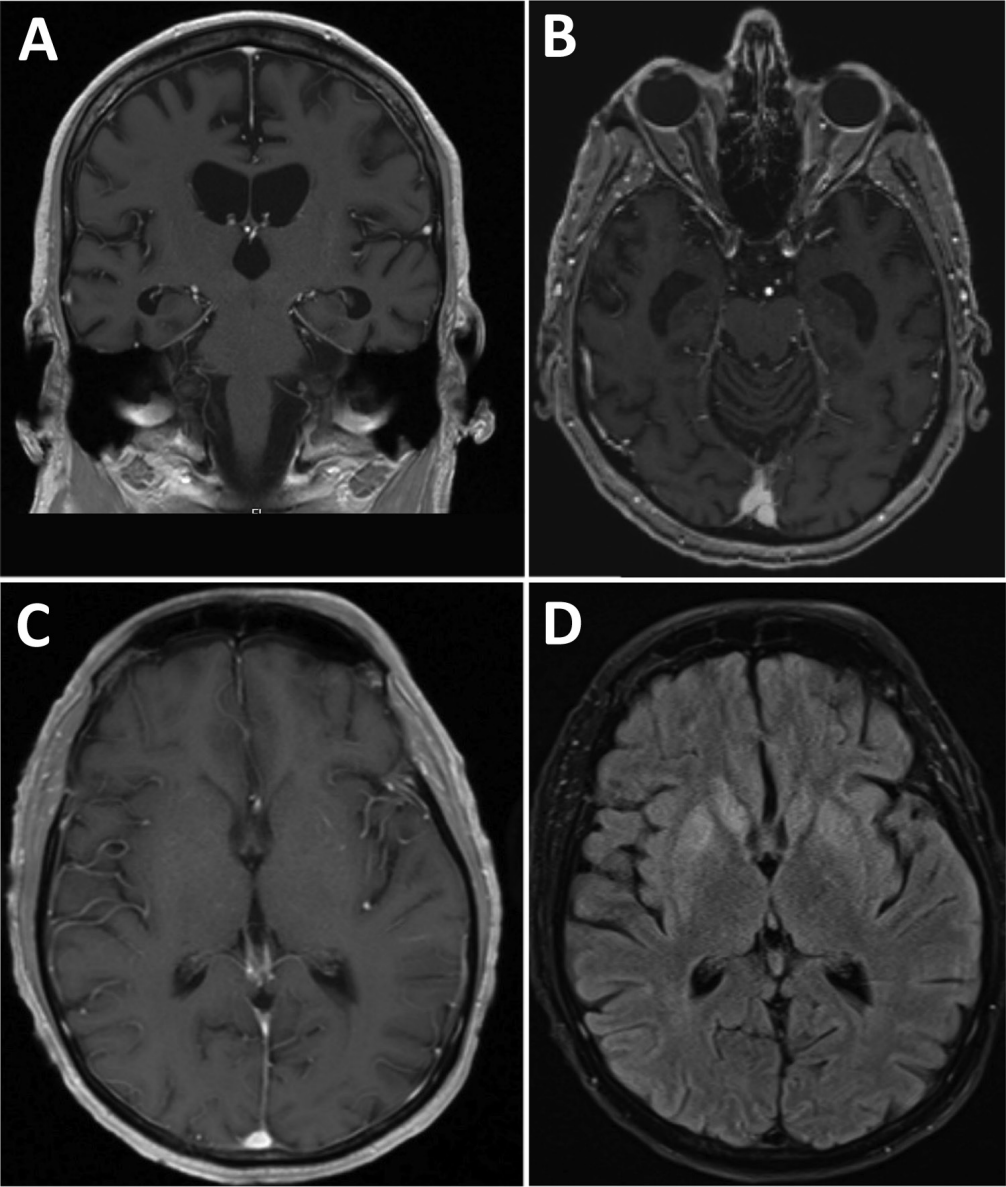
Brain magnetic resonance imaging scans from 2 patients with bunyavirus-associated meningoencephalitis, United States, 2020–2023. A, B) Case-patient 1 brain T1 postcontrast images of coronal (A) and axial (B) sections showing moderately enlarged ventricles and cerebral atrophy. C, D) Case-patient 2 brain T1 postcontrast (C) and T2 postcontrast fluid attenuated inversion recovery (D) images demonstrating bilateral basal ganglia hyperintensities with no contrast enhancement.
